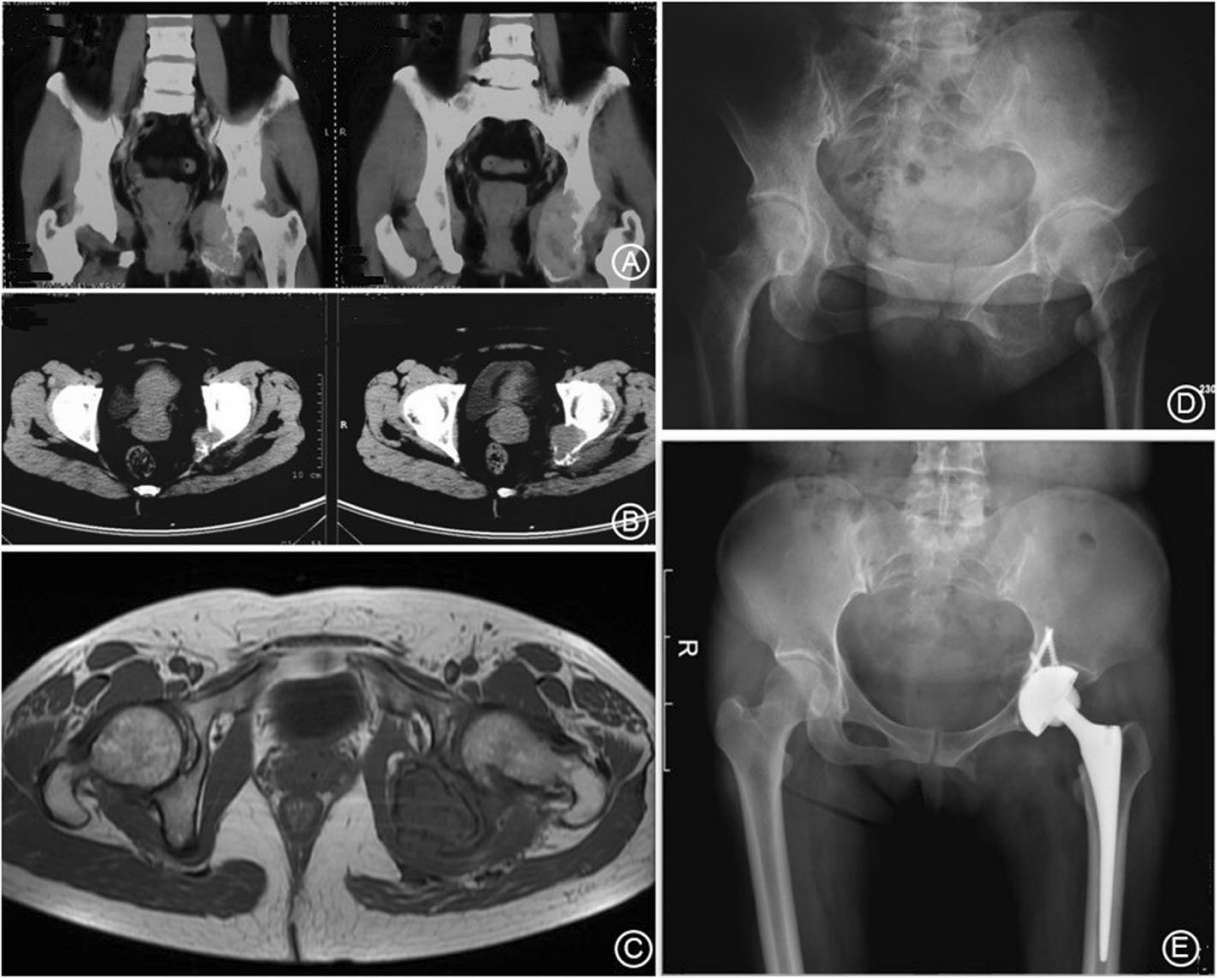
A 37y female patient diagnosed with Type B tumor (chondrosarcoma). a. Preoperative coronal CT shows a tumor with left posterior column of the acetabulum involved; b. Preoperative cross-sectional CT shows the tumor with posterior edge of the acetabulum involved; c. Preoperative MRI shows low signal on the posterior edge of acetabulum; d. Preoperative pelvic X-ray examination shows a tumor with left posterior column of the acetabulum involved; e. 1y postoperative pelvic X-ray shows the reconstruction and prosthetic position
